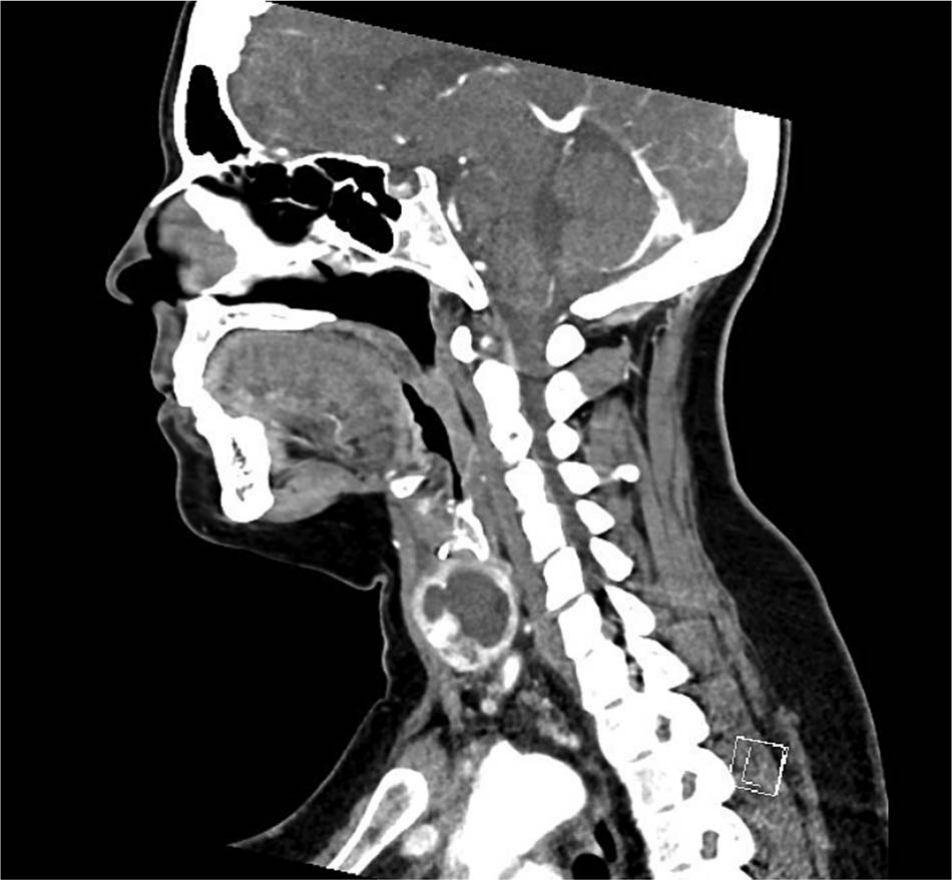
Sagittal portal venous phase CT of the neck demonstrating the cystic lesion in the left thyroid gland.
